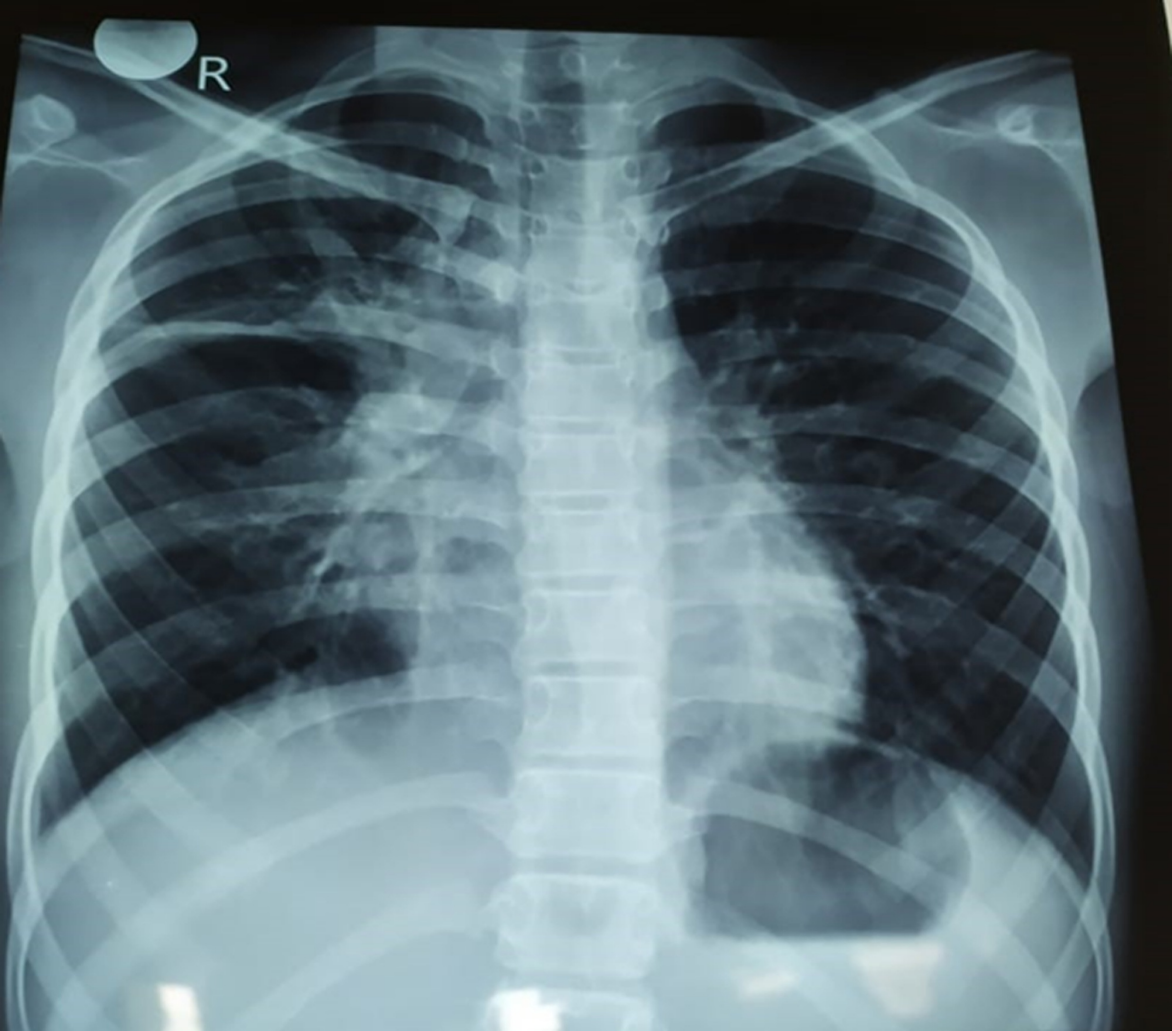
Chest X-ray showing right middle lobe consolidation
